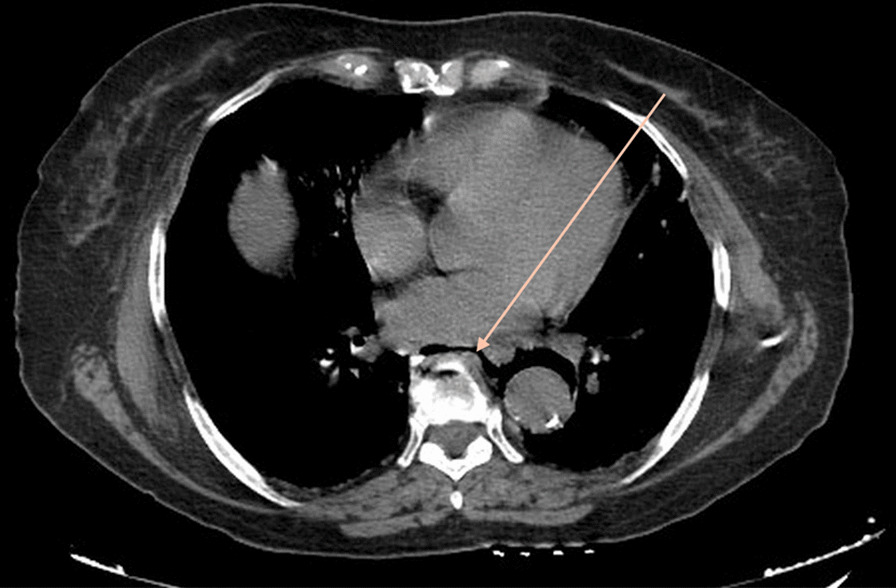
CT scan axial view showing pneumomediastinum. Arrow points to pneumodiastinum
